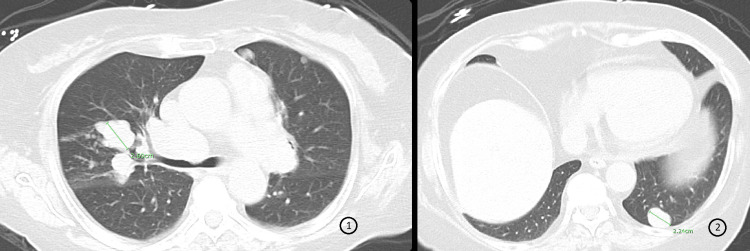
CT of the chest demonstrating multiple nodules in the lungs consistent with metastatic disease progression. *Green lines*: Identified lung parenchymal nodules measuring 2.60 cm (panel 1) and 2.24 cm (panel 2) in greatest diameter.
